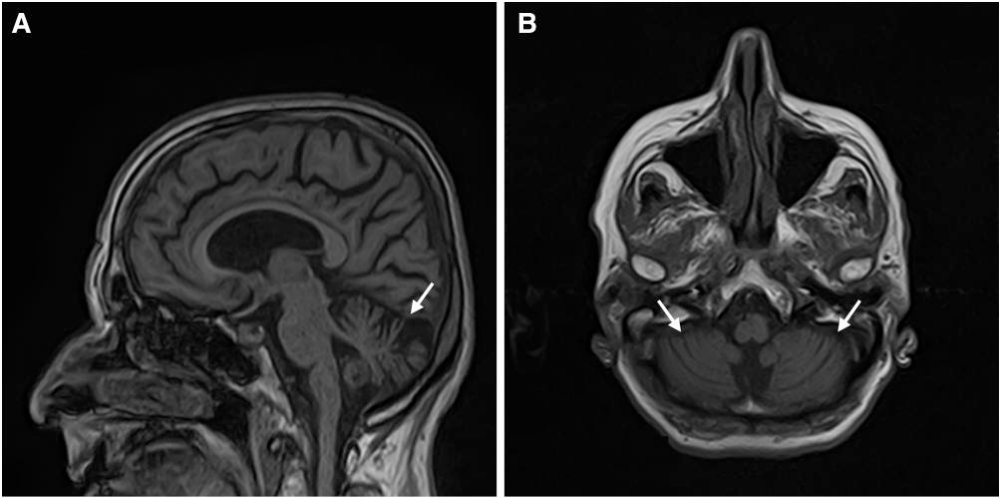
Brain MRI findings of a patient with the *FGF14* GAA repeat expansion. Moderate cerebellar atrophy (white arrows) in a female patient (P11) with SCA27B, (A) in sagittal T1 and (B) axial T1 views.
